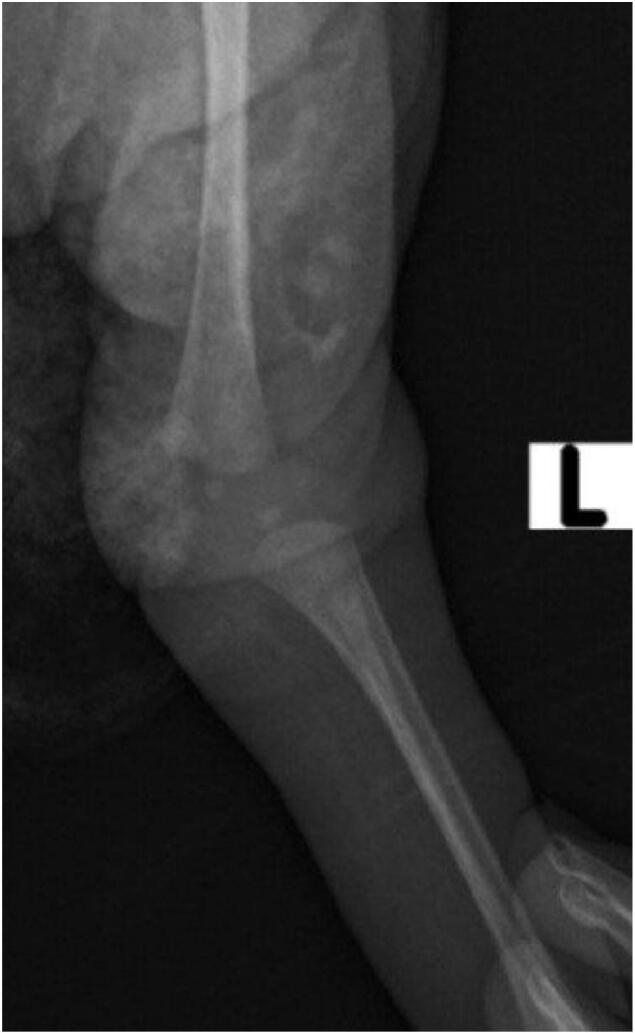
Initial left knee AP X-ray showing left knee dislocation.
